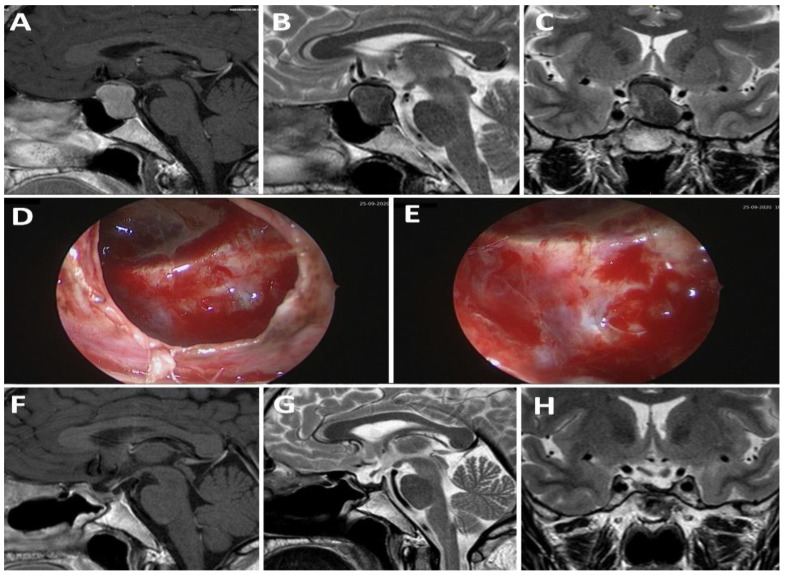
A 43-year-old patient presenting pituitary apoplexy in the context of a non-functioning macroadenoma. (A–C): Preoperative MRI; (D,E): intraoperative findings; (F–H): postoperative MRI.
